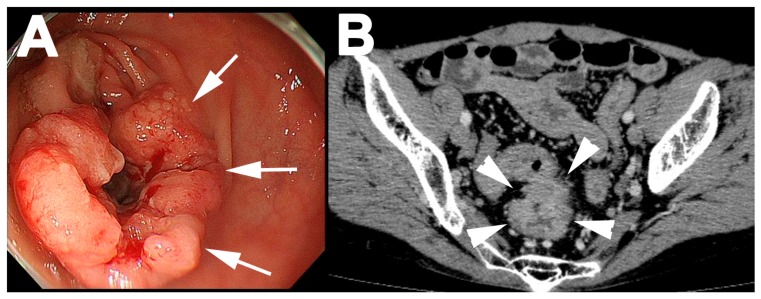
Endoscopic and radiographic features of rectal cancer. (A) Endoscopic examination showed type 2 cancer (white arrows); (B) Axial computed tomography scans showed the tumor (white arrowheads) in the rectum.
